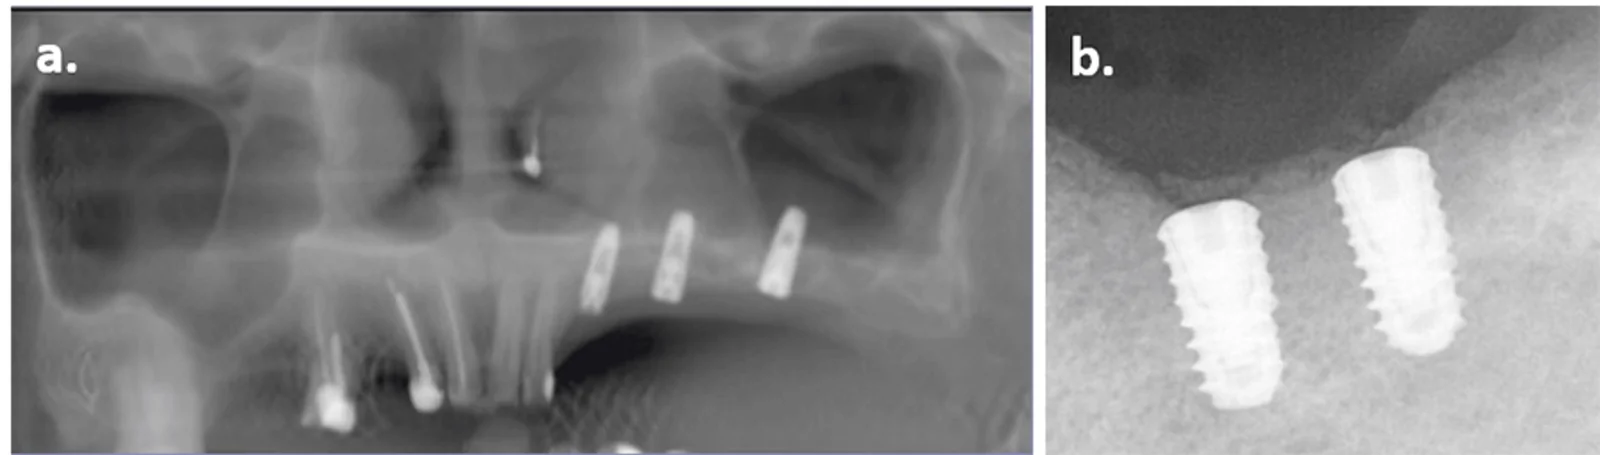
Postoperative X-ray images taken after six months show radiographically stable implants. (a) Orthopantomogram (OPG) image of maxillary implants placed at 23, 25, and 27 sites. (b) Radiovisiography image of mandibular implants placed at 36 and 37 sites.
